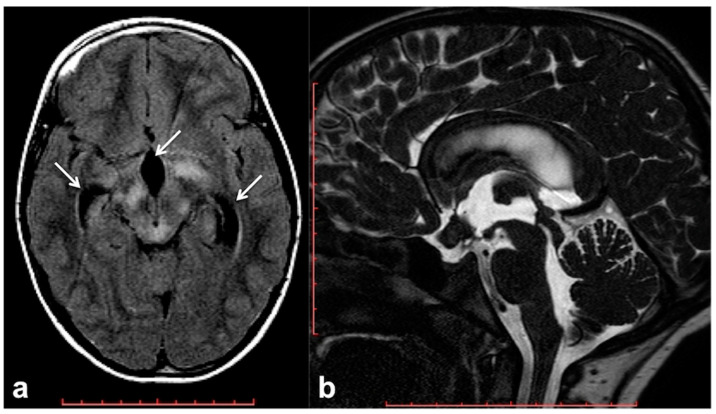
Brain MRI of a 6 year-old boy with NF1 and aqueductal stenosis: axial fluid attenuation inversion recovery (FLAIR) (a) showing bilateral basal ganglia and brainstem hyperintensities consistent with FASI. Supratentorial hydrocephalus with enlarged third ventricle and temporal horns of the lateral ventricles was also seen (white arrows); no transependymal CSF flow in periventricular areas was visible, and the fourth ventricle was normal. Sagittal 3D heavily T2-weighted sequence (b) revealed the presence of a thickened web in the cerebral aqueduct causing stenosis and hydrocephalus.
